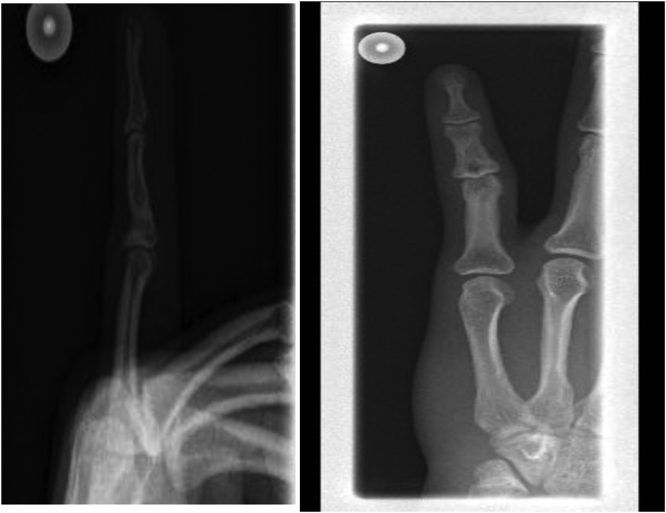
X-rays of the left little finger demonstrating significant interval improvement of the osteomyelitis in the middle phalanx after TB therapy.
